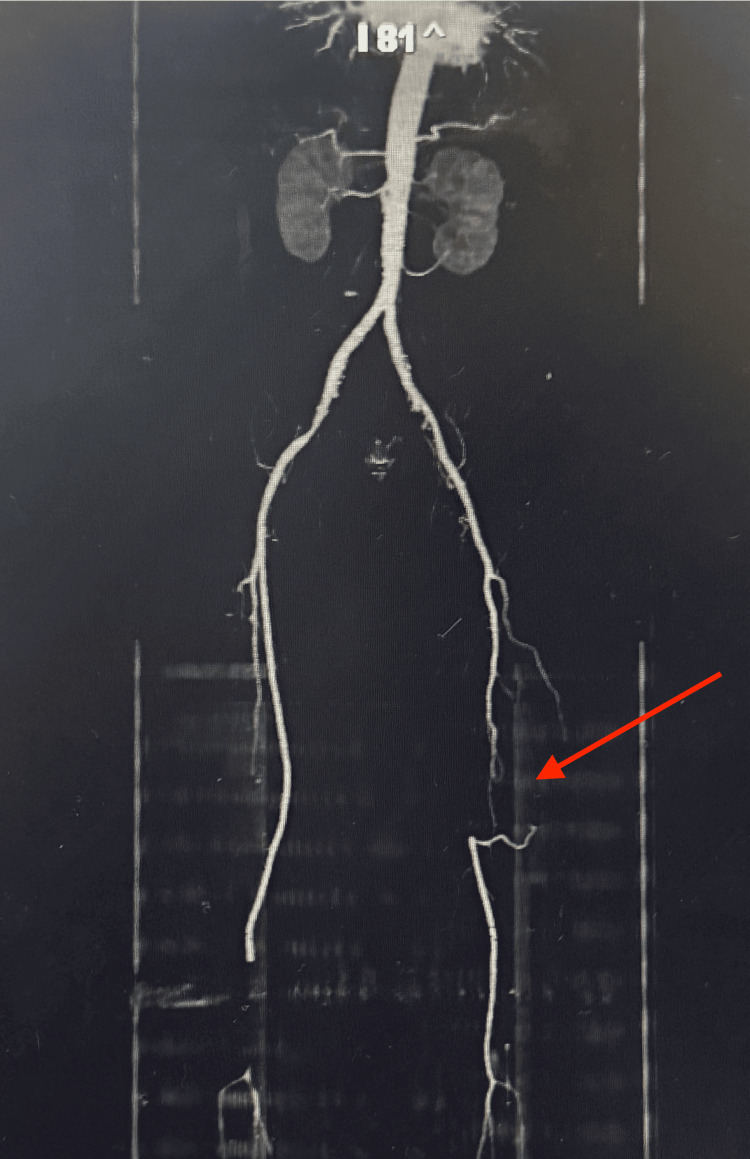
CT angiogram with runoff demonstrating complete occlusion of the left SFA (red arrow) SFA, superficial femoral artery
